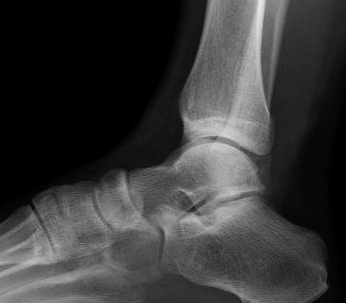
Plain radiograph of the left foot. The radiograph demonstrates a large lytic lesion of the left cuboid.
